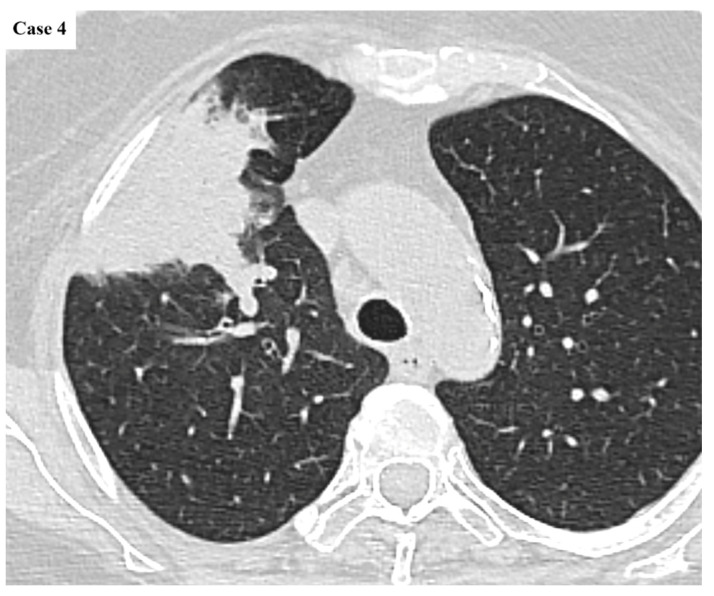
Axial CT image of a lung mass found peripherally in the anterior segment of the right lung.
